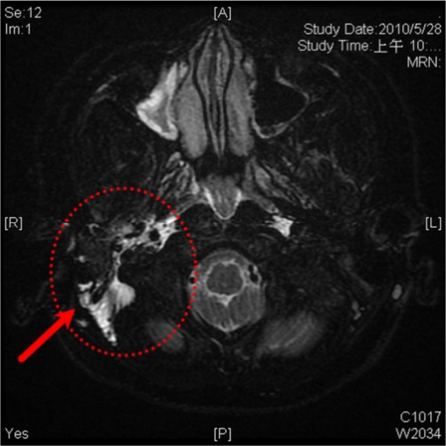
MRI showed CSF accumulation at right middle ear
